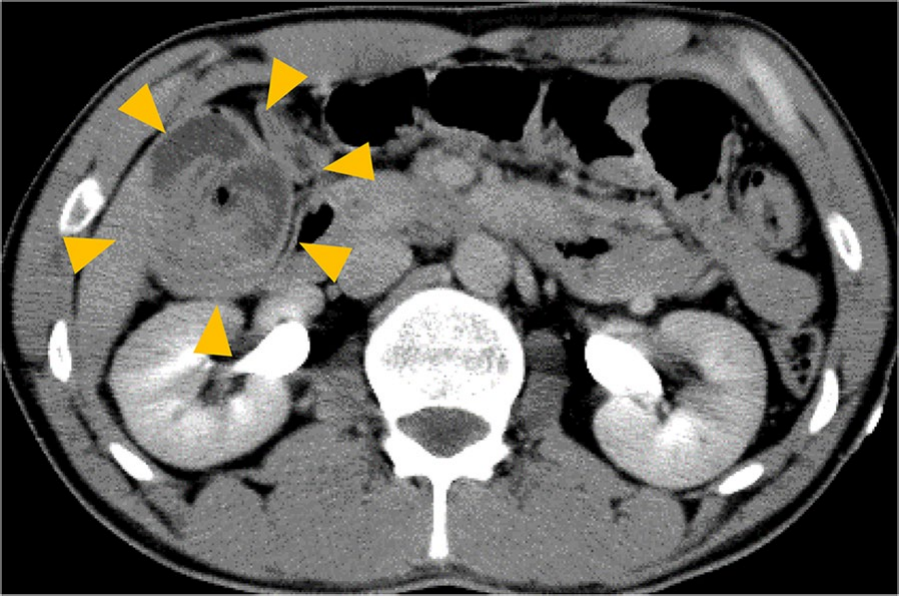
Preoperative contrast-enhanced computed tomography. The target sign was observed in the transverse colon. Distinct anatomic features are clearly seen, such as the entering wall, mesenteric fat and vessels, returning wall, and intraluminal space (yellow arrow)
